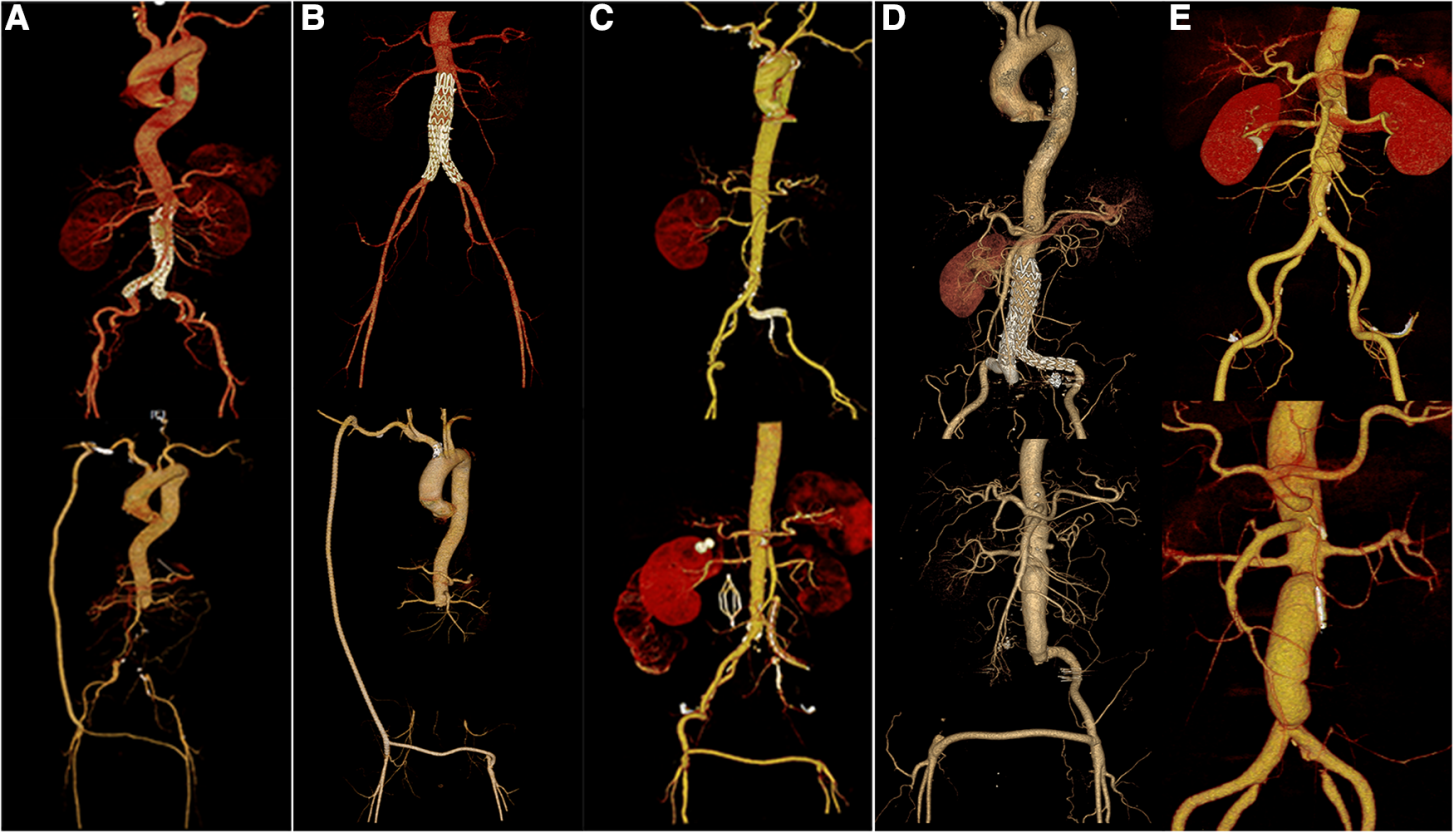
(A–E). Preoperative and postoperative CTA data of patients 1–3, 5, and 6, respectively.
